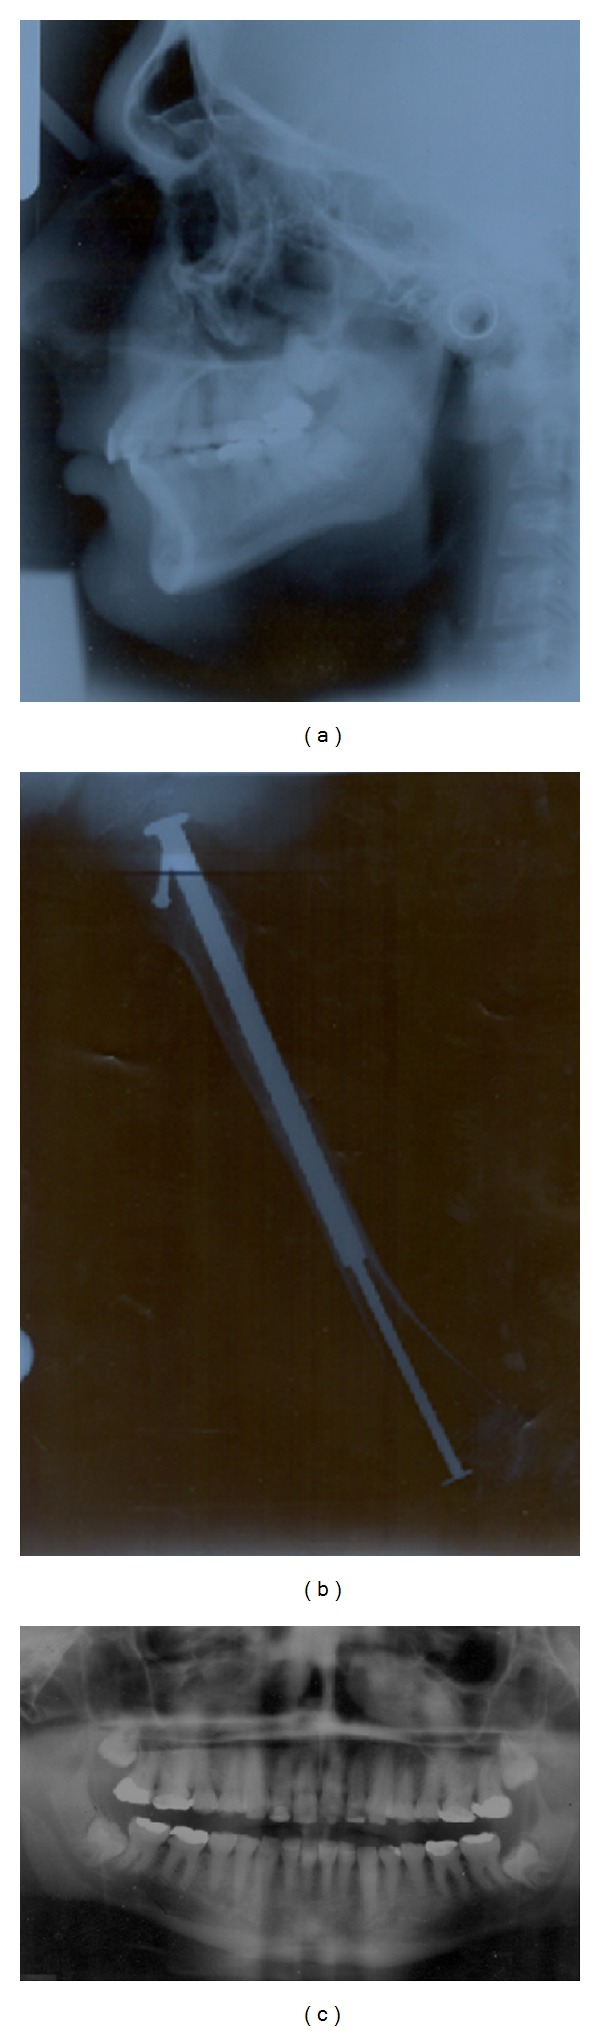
Patient radiographs: Lateral cephalograph (a); plain femoral radiograph (b); and orthopantomograph (OPG) (c).
